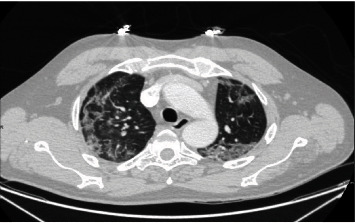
Axial contrast-enhanced chest CT in lung window showing bilateral peripheral ground-glass opacities.
